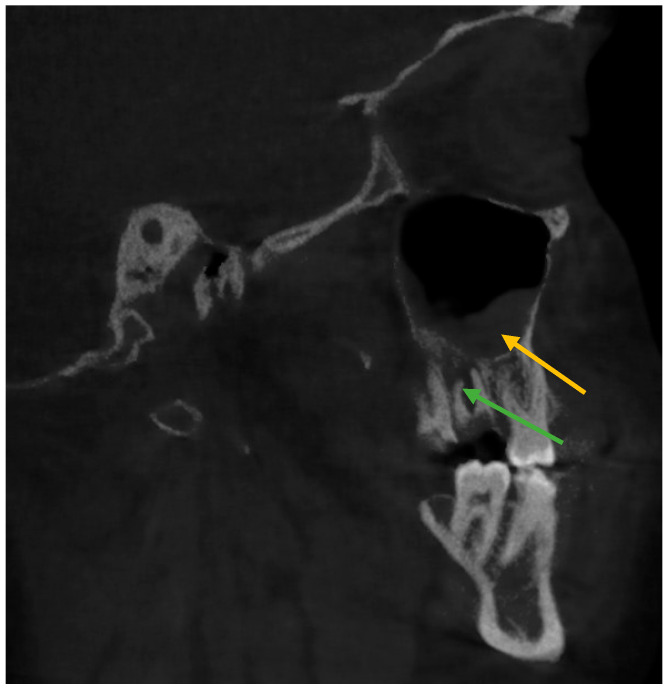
Changes in mucosal thickness in maxillary sinus observed in sagittal view on CBCT imaging. (Yellow arrow shows mucosal thickening, green arrows show periapical infection).
